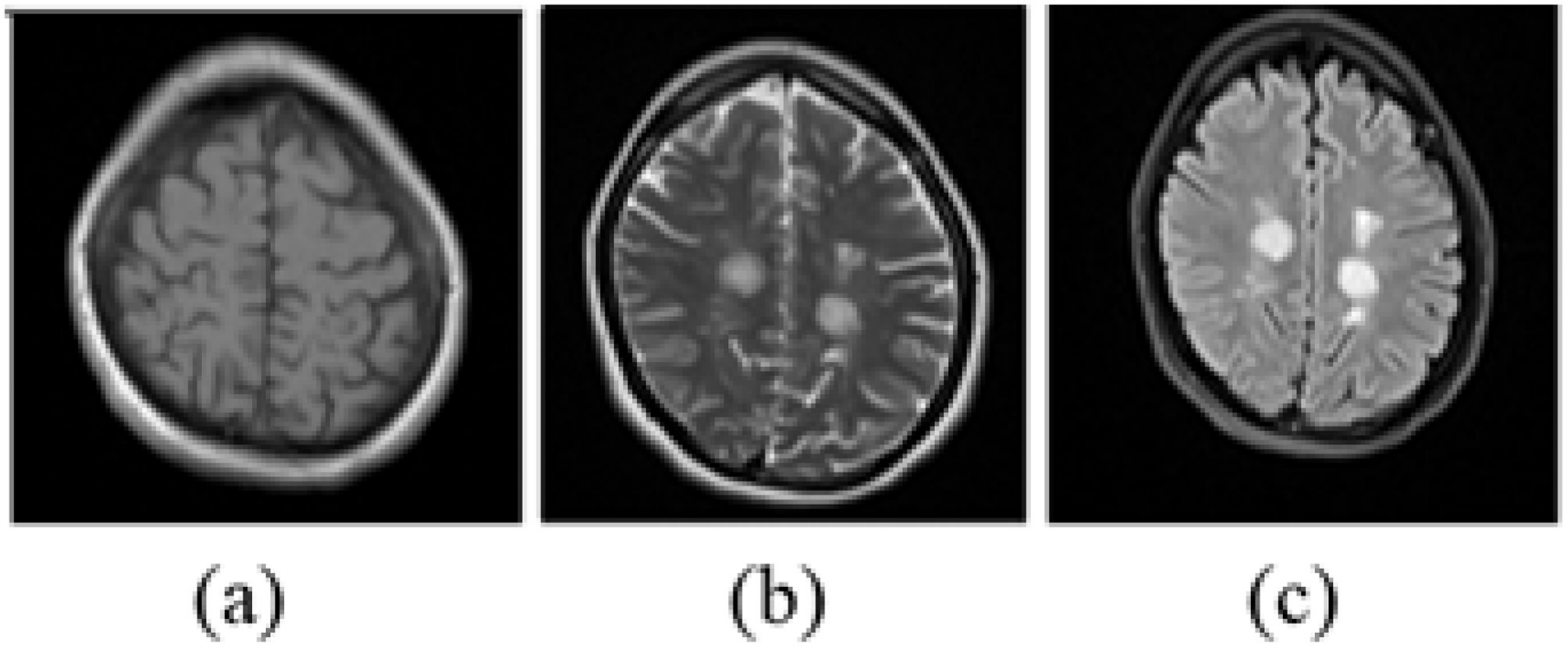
Mendeley dataset sample brain MRI in different image modalities when slice number is 15 for the second patient in the dataset. (a) T1-weighted, (b) T2-weighted. (c) FLAIR.
